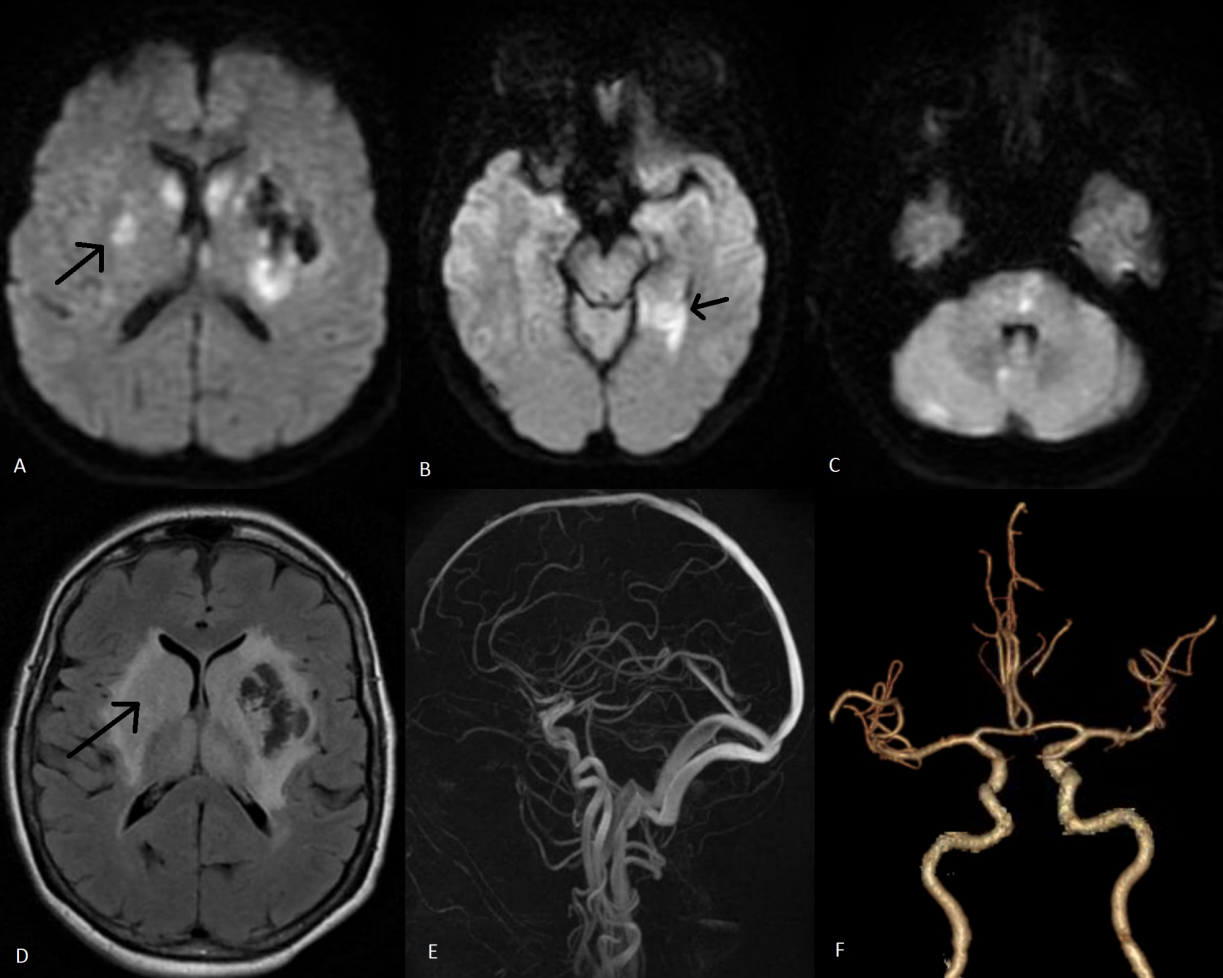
Magnetic resonance imaging brain and vascular studies done on postoperative day 5 (A-C) Diffusion-weighted magnetic resonance imaging shows multiple infarcts via patchy areas of diffusion restriction; (D) Fluid-attenuated inversion recovery signal changes in the bilateral basal ganglia; (E-F) Magnetic resonance venography and computed tomography angiography, respectively, show grossly normal vascular studies
